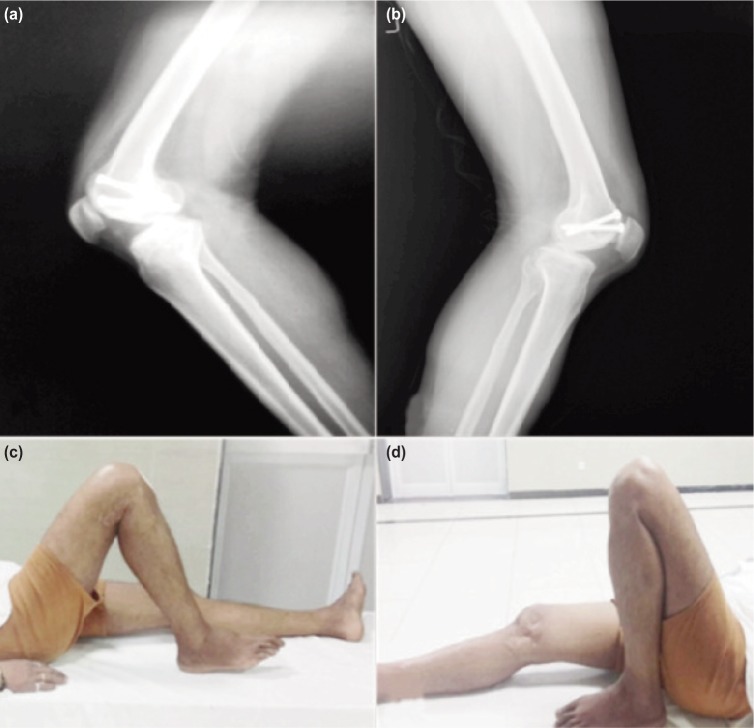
Radiographs at 36 month follow up for (a) right and (b) left knee. Functional outcome by the end of 36 month were ROM in (c) right knee was 0-125° and in (d) left was 10-160°.
